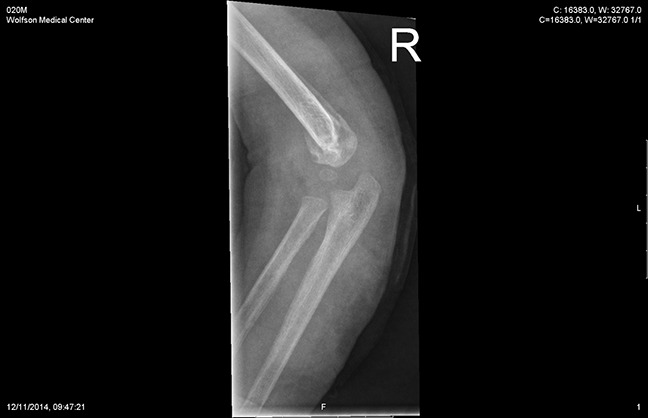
Radiograph after Kirschner wire removal
